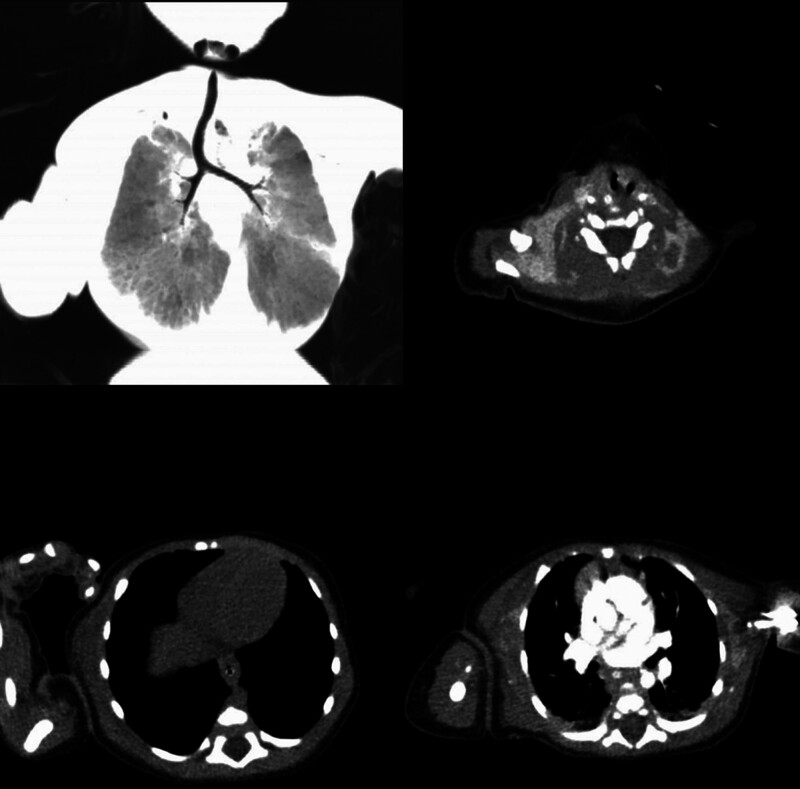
CT images of the child. The CT results show blurry images in part of the tissue structures and interstitial spaces of the neck and chest, along with soft tissue edema and air accumulation. The upper tracheal segment was tortuous and irregular. The above findings are consistent with pneumonia in accompany with partial pulmonary consolidation and air retention. Besides, multiple segmental bronchi are vaguely outlined, with narrowed bronchial lumen observed.
